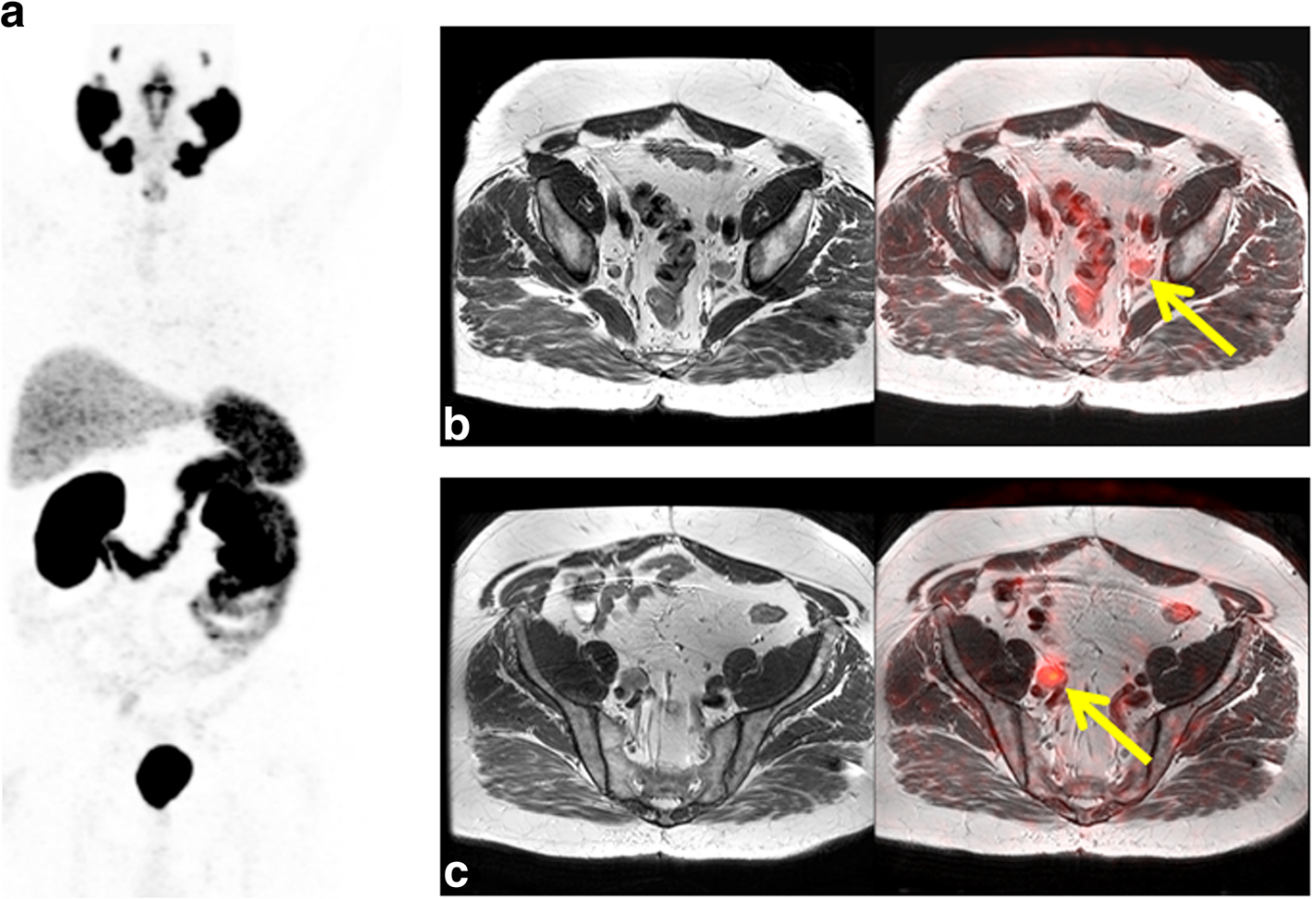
Patient 8. A 58-year-old-patient with PSA relapse (PSA level 4.44 ng/ml) after radical prostatectomy. MIP (a), MR and ^68^Ga-PSMA PET/MR (b-c) images showing pelvic lymph node metastasis (yellow arrows)
